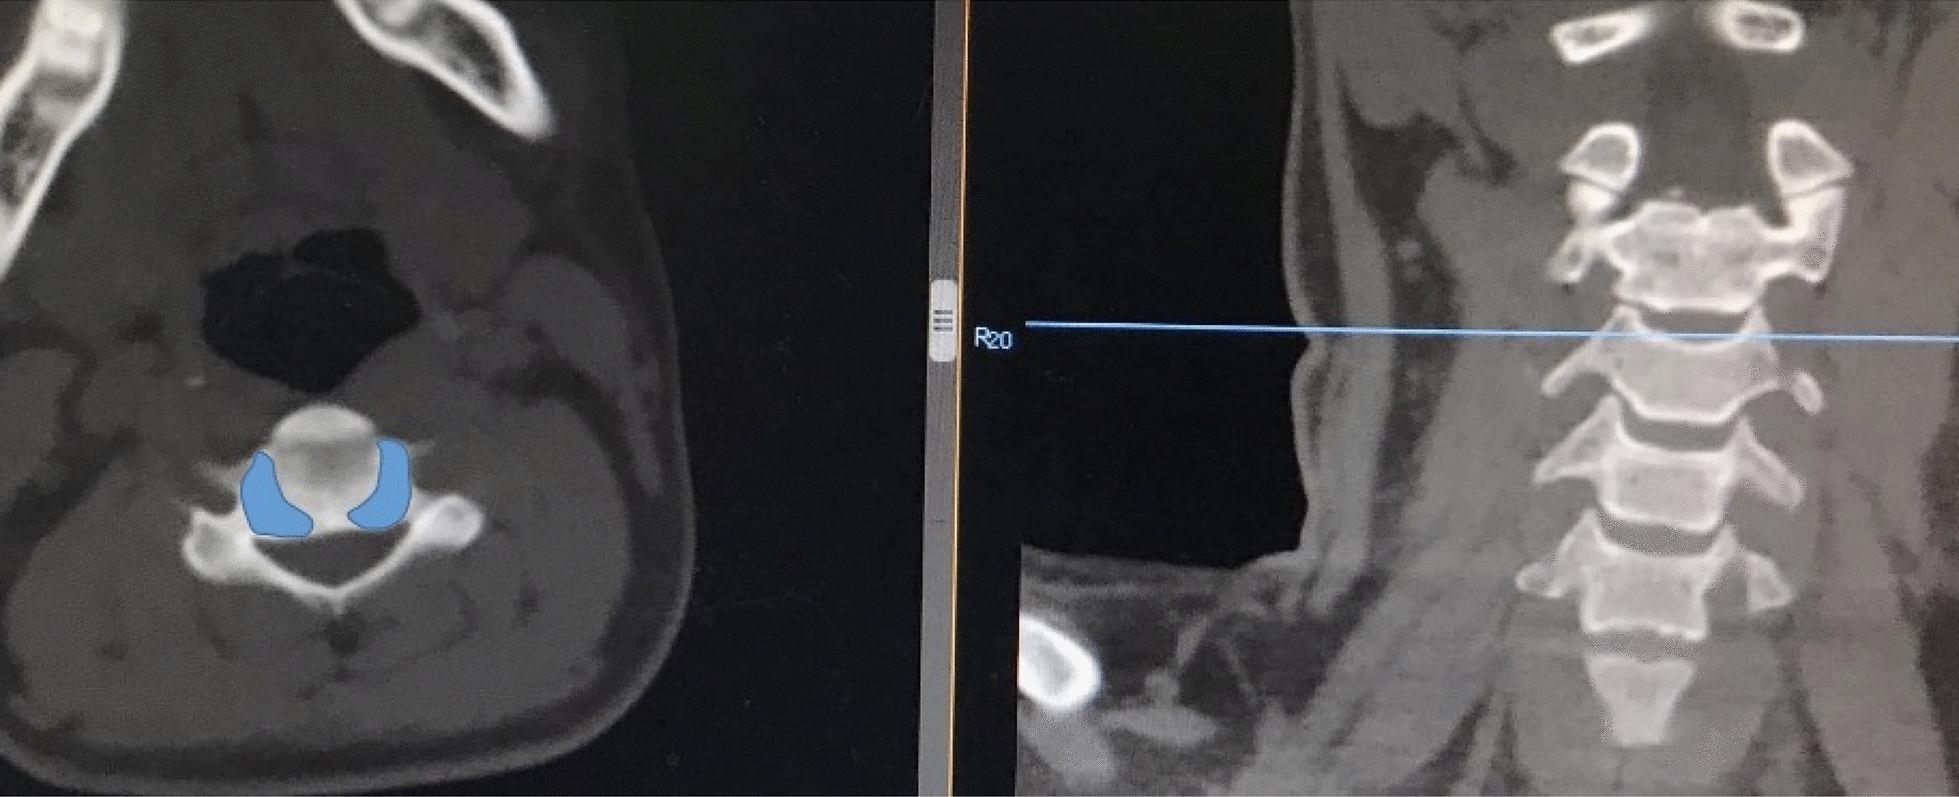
The uncovertebral joints were observed fully on CT
